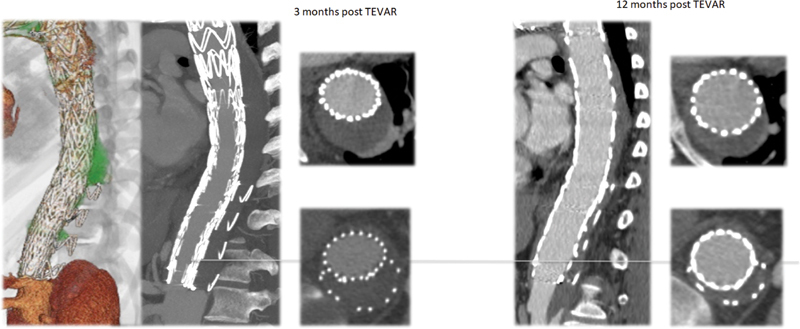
Computed tomography (CT) post- thoracic endovascular aortic repair and “candy plug” CT scan showing effective resolution of Type B aortic dissection.
